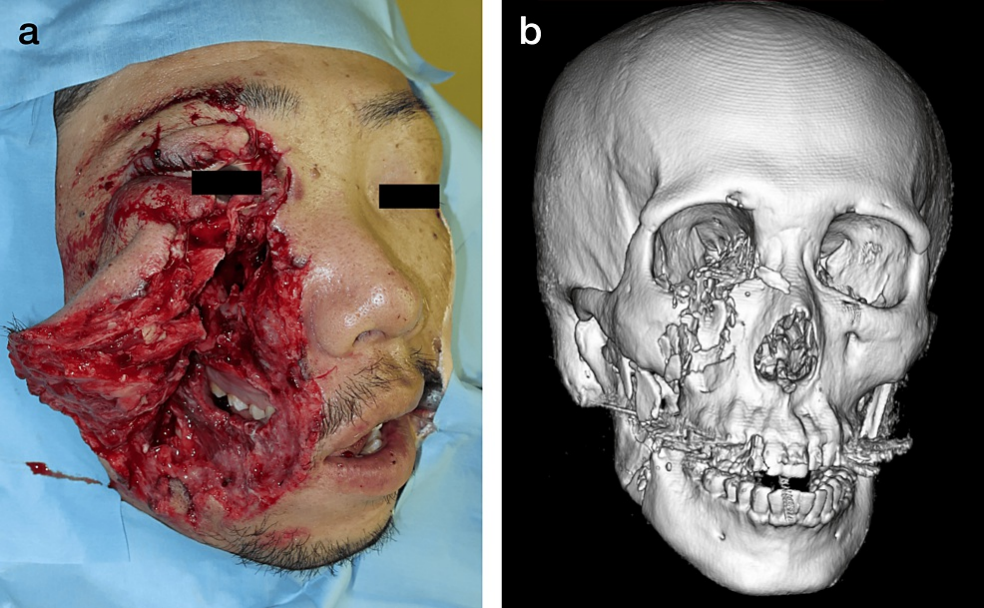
The nature of the maxillofacial trauma A clinical photograph shows deep lacerations of the right eyelid, medial canthus, cheek, and jaw with venous bleeding (a). Three-dimensional computed tomography of the head shows multiple fractures of the right frontal bone, zygomatic bone, maxilla, and mandible (b).
